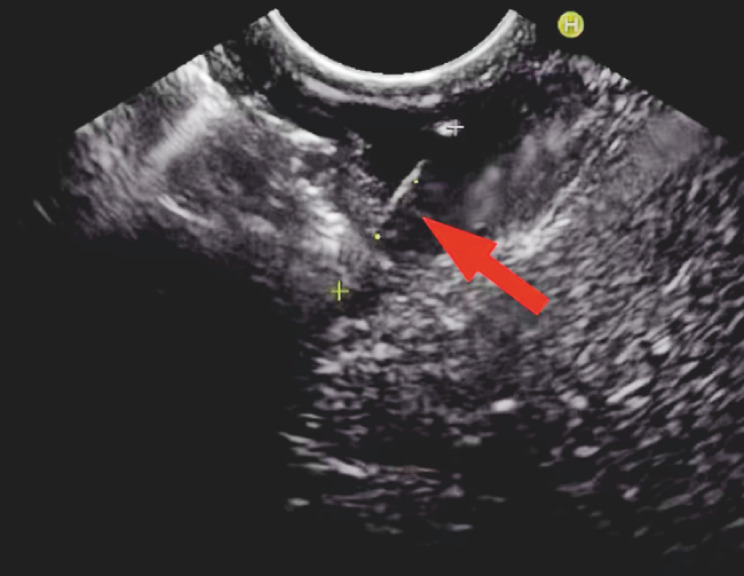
Endoscopic ultrasonography revealed the location and depth of a fishbone (arrow).
